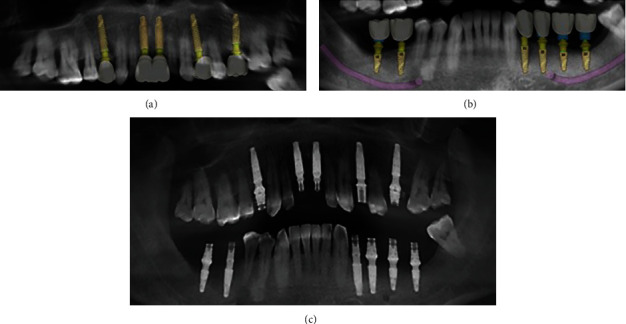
Virtual planning of implants and abutments (a, b) and immediate loading panoramic radiography (c).
